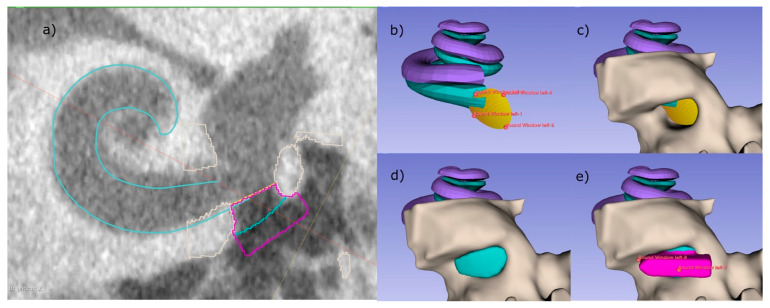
RNI creation using the developed semi-automated software as 3D Slicer™ plugin. (a) A CBCT slice of the basal turn of the scala tympani (cyan) with the implant including handle (pink) sitting inside the RWN. (b–e) 3D visualization of the individual steps, that are also visible as outlines in (a). (b) Four control points are placed, defining the RWM (yellow) and limiting the backside of the implant body. (c) The bone (light gray) surrounding of the RWN is segmented by thresholding, allowing the RWN to be filled (cyan, d). In a last step, a handle is added to the implant (pink), helping to define the orientation of the implant.
